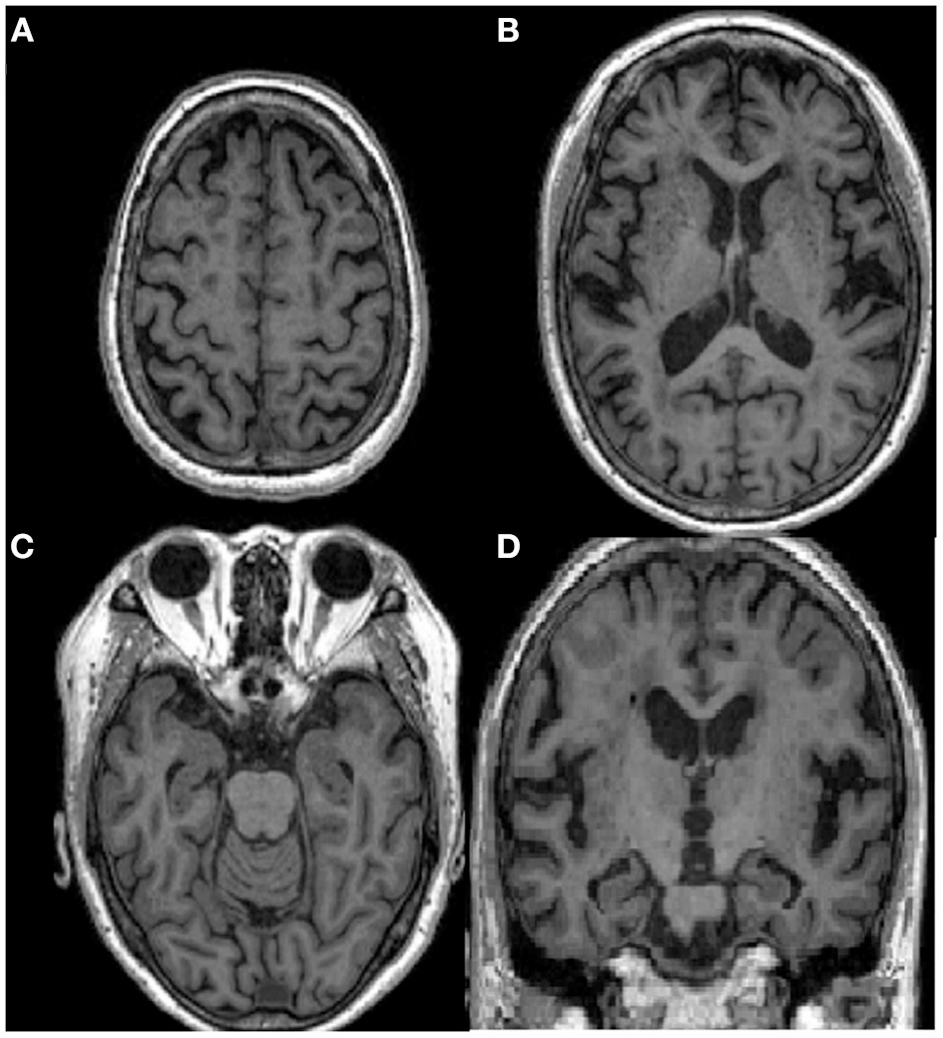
Brain MRI of the patient showing mild diffuse atrophy, compatible with her age. (A–C) Axial sections of T1 weighted images; (D) A coronal section.
